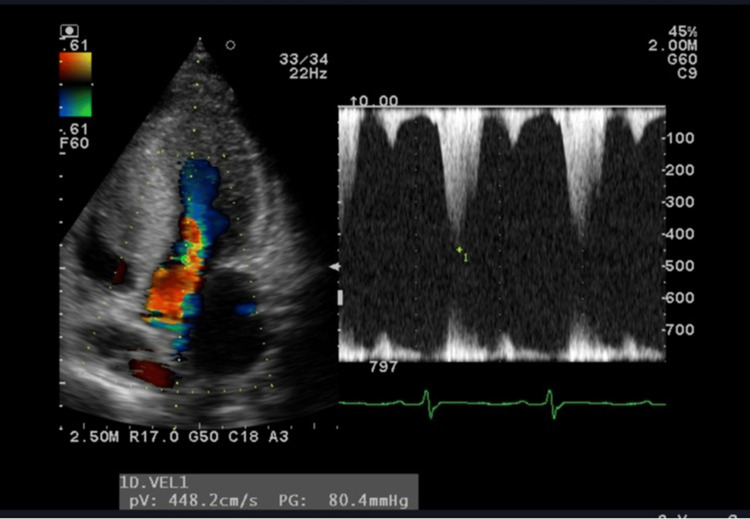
Echocardiography early in the first pregnancy The left ventricular outflow tract pressure gradient was as high as 80 mmHg.
